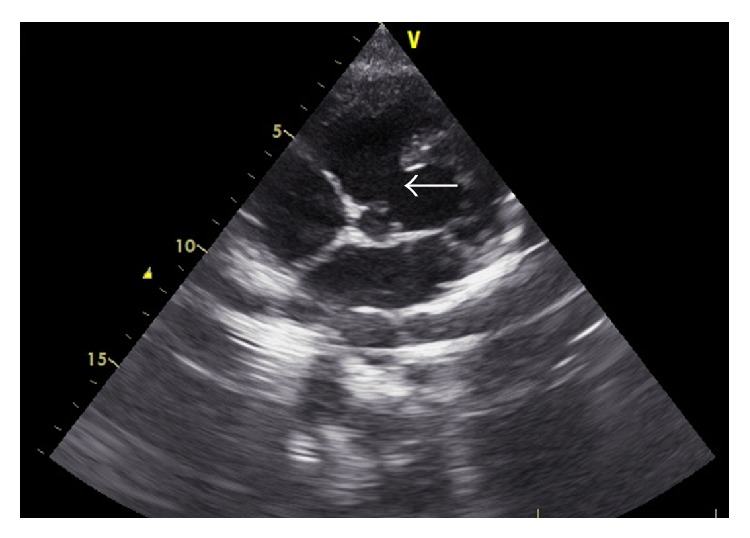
Transthoracic echocardiography: 5-chamber view shows a large malaligned nonrestrictive subaortic VSD (thin arrow).
